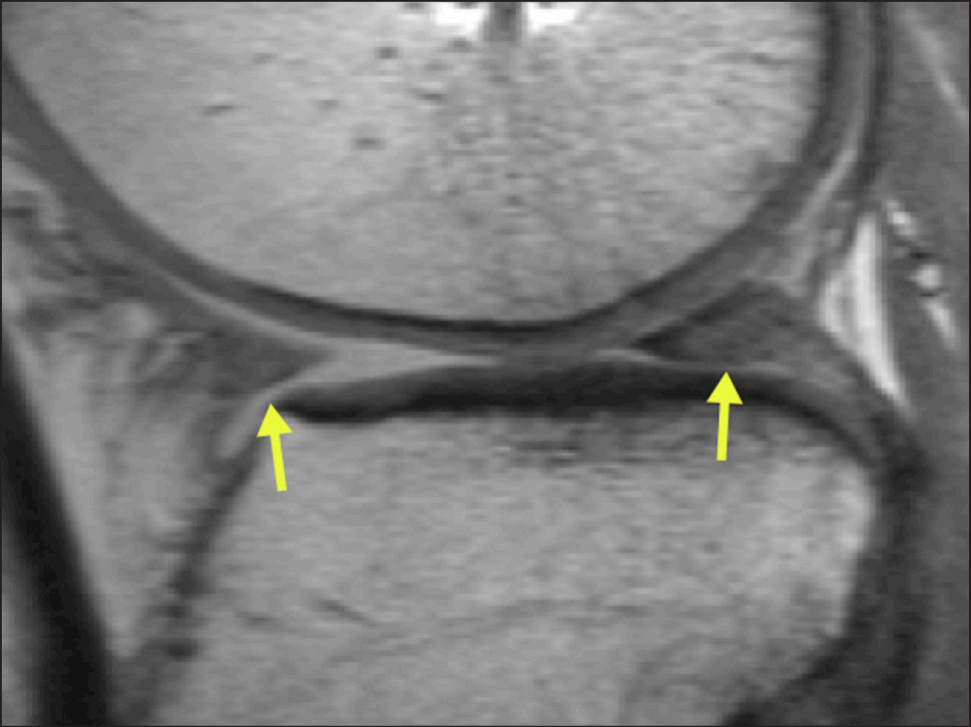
Sagittal T1-weighted fast spin echo MR-image of a lateral meniscal allograft. The allograft demonstrates generalized high signal (yellow arrow). These signal changes are the result of a change in water content and do not necessarily have to be a sign of degeneration.
